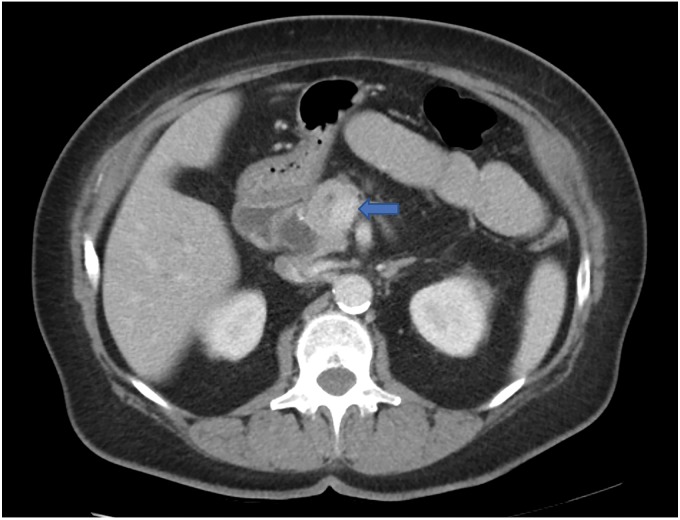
Axial CT scan of the abdomen in case 2 with intravenous contrast showing an ill-defined pancreatic head mass (arrow) that measures 3.6 × 3.6 cm. There is associated pancreatic ductal dilatation with abrupt cutoff at the level of the mass. The mass abuts the superior mesenteric vein and there is attenuation of the main portal vein and SMV at the confluence related to the mass. SMV, superior mesenteric vein.
